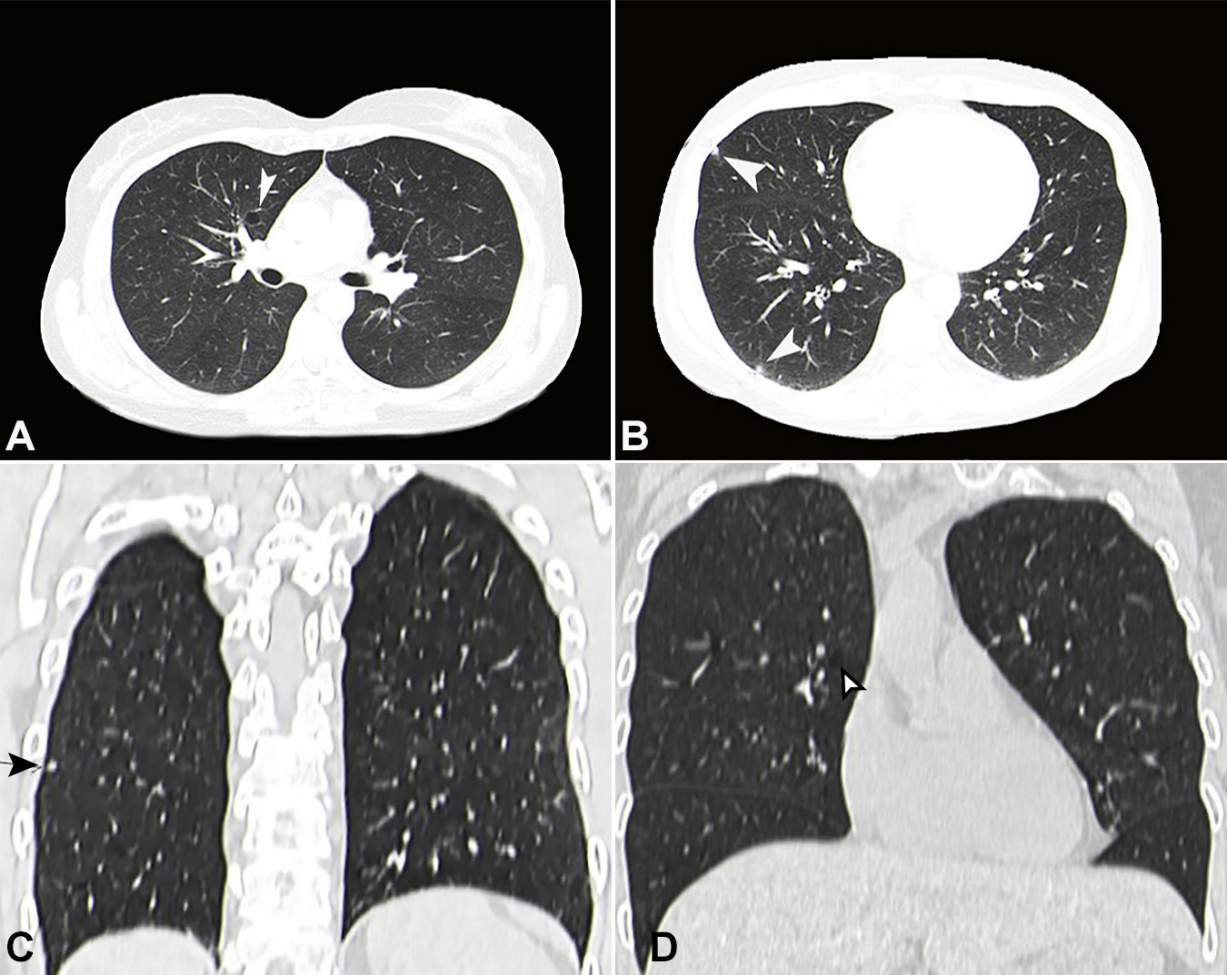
Chest CT scan, A and B – axial plane- with tiny pulmonary nodules and cysts (arrows) involving both lungs, likely due to pulmonary Langerhans cell histiocytosis. C and D - Coronal plane - showing pulmonary cysts and nodules.
